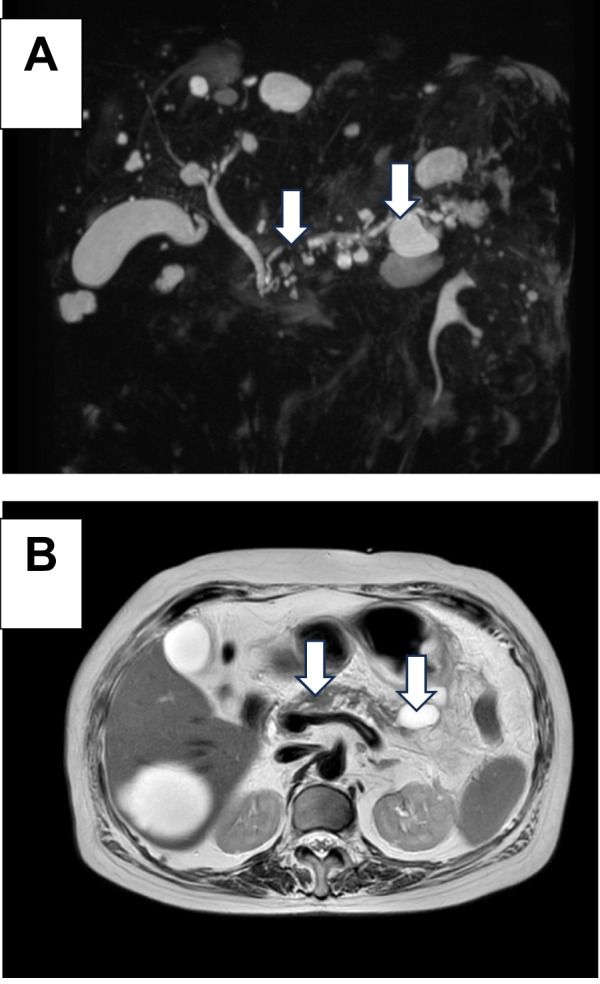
Magnetic resonance cholangiopancreatography findings. A, B Magnetic resonance cholangiopancreatography revealed a tumor in the pancreatic body and complete obstruction of ~ 12 mm of the main pancreatic duct. A high-signal pancreatic effusion and pseudocyst were seen on a T2-weighted image of the pancreatic tail (white arrow)
